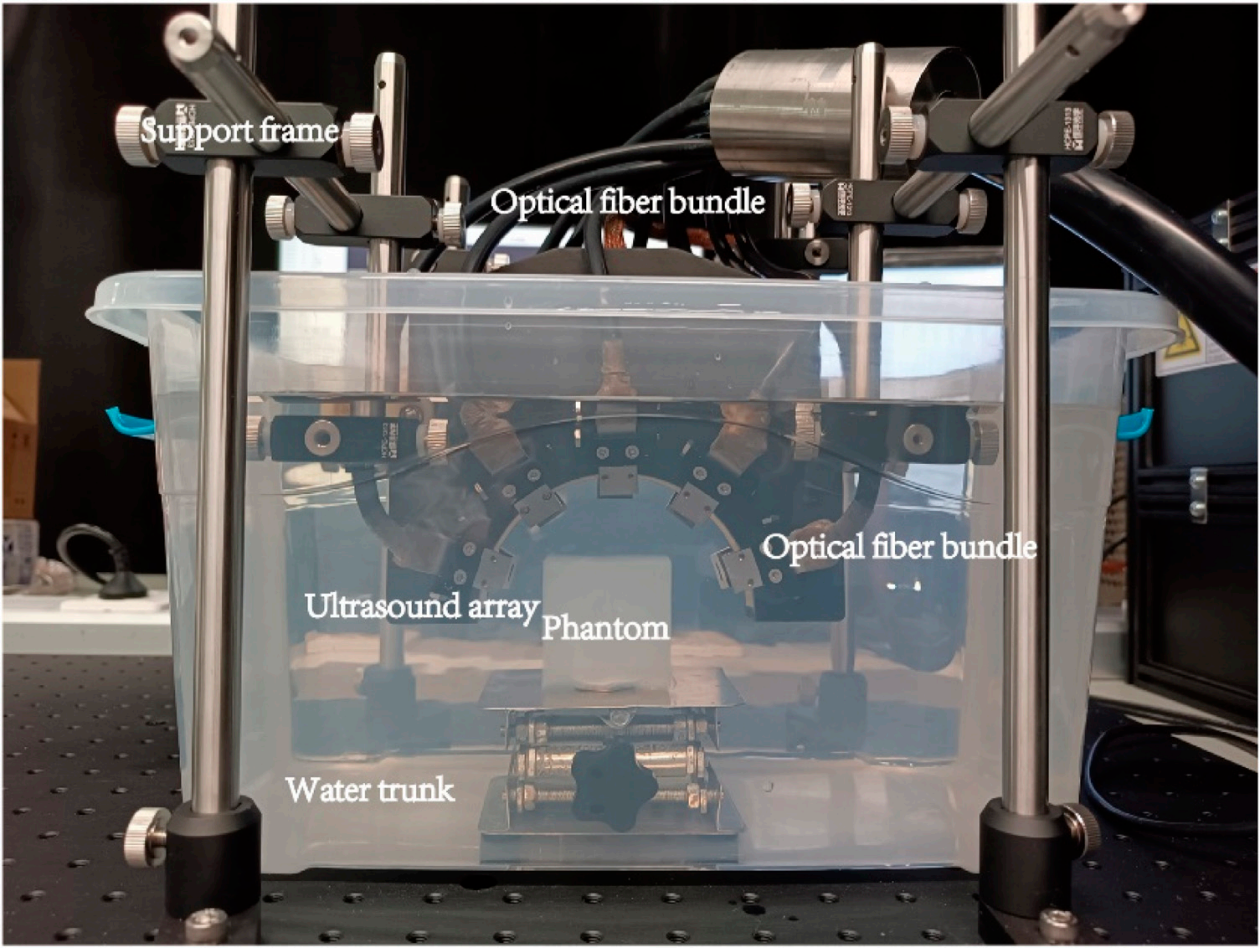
Ultrasonic probe and light source physical arrangement.
